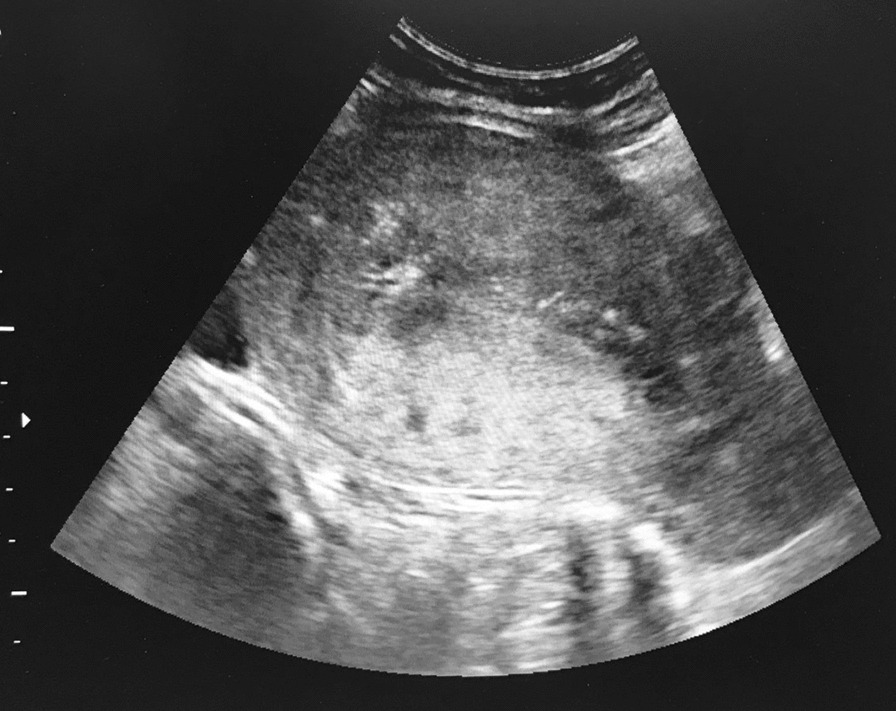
Dilatation and curettage under ultrasound guidance
